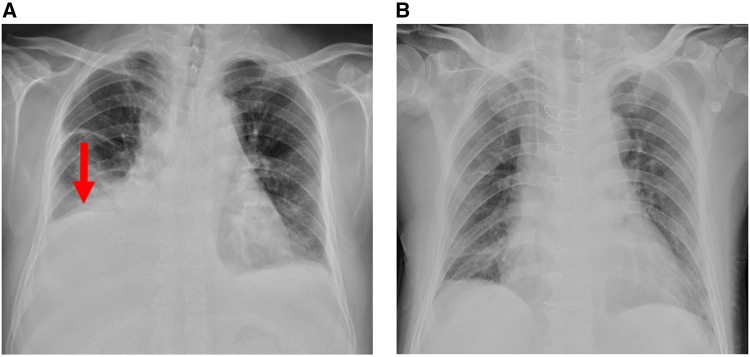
The chest X-ray on admission (*A*) showed high density involving both lungs symmetrically and massive pleural effusion, and chest X-ray (*B*) after pericardectomy indicated significant improvement. Red arrow indicates pleural effusion.
